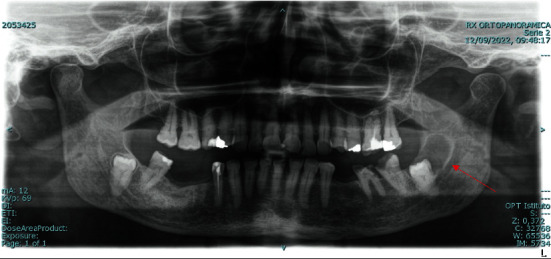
Panoramic radiograph: a follicular cyst was identified at element 3.8 (red arrow).
